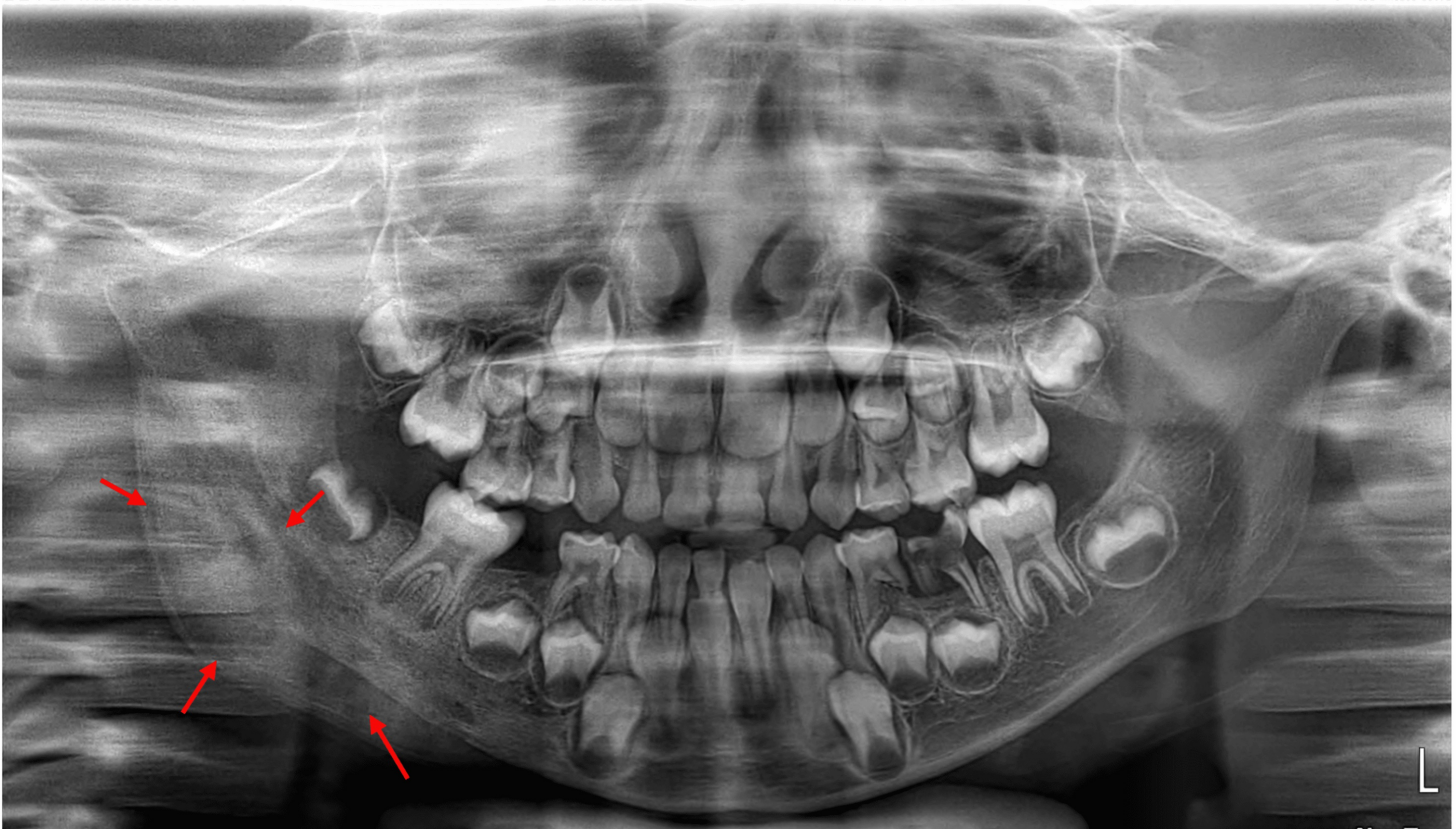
Orthopantomogram showing a radiopaque ossified lesion in the right mandible (red arrows).
